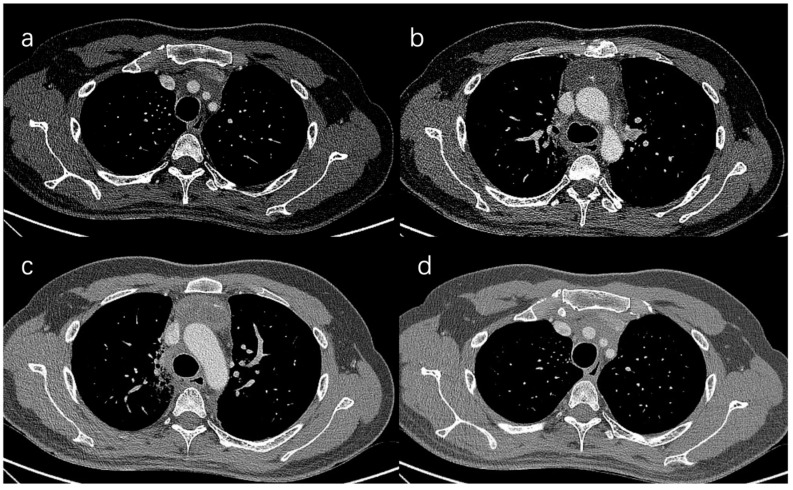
CT scan of 32-year-old patient with thymoma. (a) The relationship of tumor to the top of SVC, which is tumor free. The left innominate vein joins the SVC; (b) The relationship of tumor and the top of superior vena cava which is tumor free; (c) The relationship of tumor and the aorta arch which is potentially tumor free; (d) The relationship of tumor and the aorta branches, which are potentially tumor free.
